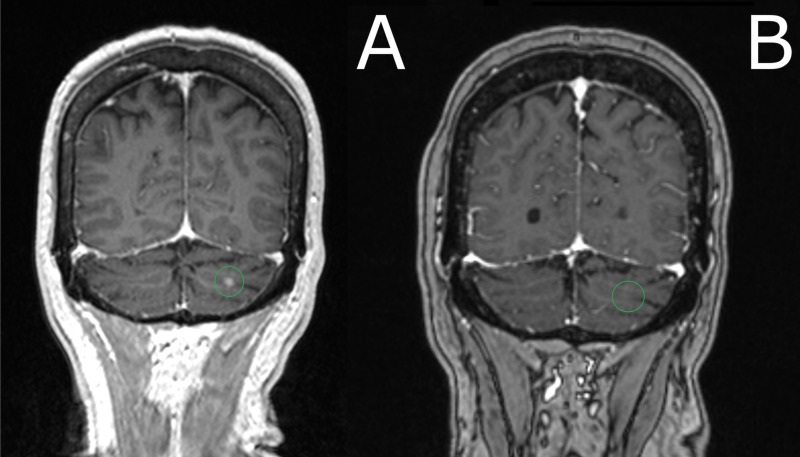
Comparison of brain MRI at presentation (a) versus 3 months after SRS procedure (b) A) MRI brain imaging revealed two contrast-enhancing lesions in the left frontal lobe and left cerebellar hemisphere (pictured here). T1 Axial image, post-gadolinium contrast), measuring 1.25 cc and 0.07 cc, respectively. Patient underwent SRS, with 22 Gy delivered to the 50% isodose line to each lesion. B) Three months after stereotactic radiosurgery (SRS), repeat MRI demonstrated resolution of the cerebellar lesion. MRI: magnetic resonance imaging; SRS: stereotactic radiosurgery
